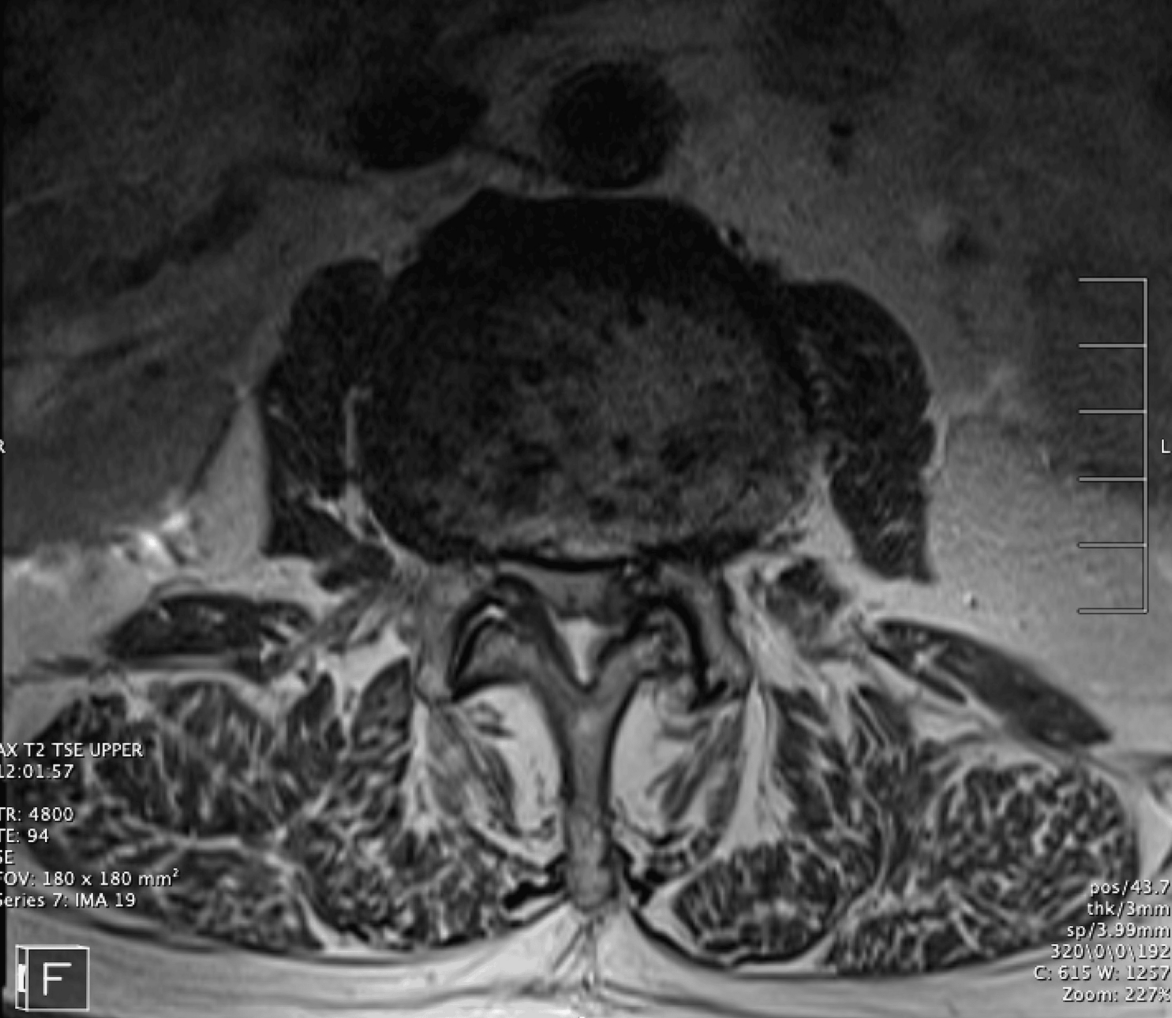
Axial lumbar MRI T2 This figure provides a detailed axial snapshot of the lumbar L4-L5 intervertebral level, highlighting the following pathological findings: posterior disc uncovering with a prominent circumferential disc bulge, particularly more pronounced on the left side; advanced facet arthropathy with joint effusions; moderate thickening of the ligamentum flavum; severe canal stenosis resulting in complete effacement of cerebrospinal fluid (CSF) around the nerve roots and in both lateral recesses; moderate right foraminal stenosis; and severe left foraminal stenosis.
